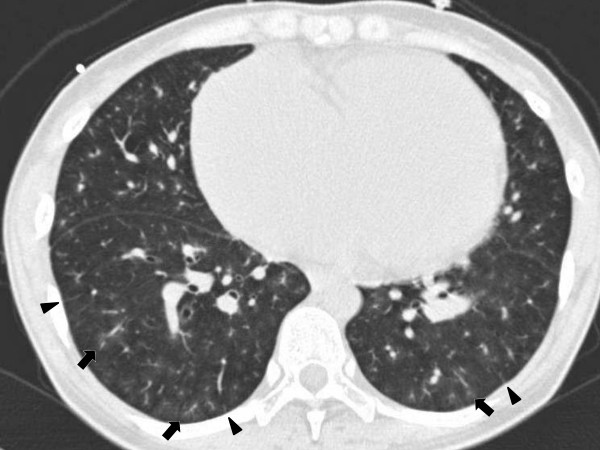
The computed tomography scan of a 25-year-old woman with severe idiopathic pulmonary arterial hypertension reveals thickening of the interlobular septa (arrowheads) and small multifocal areas of centrilobular ground-glass attenuation.
